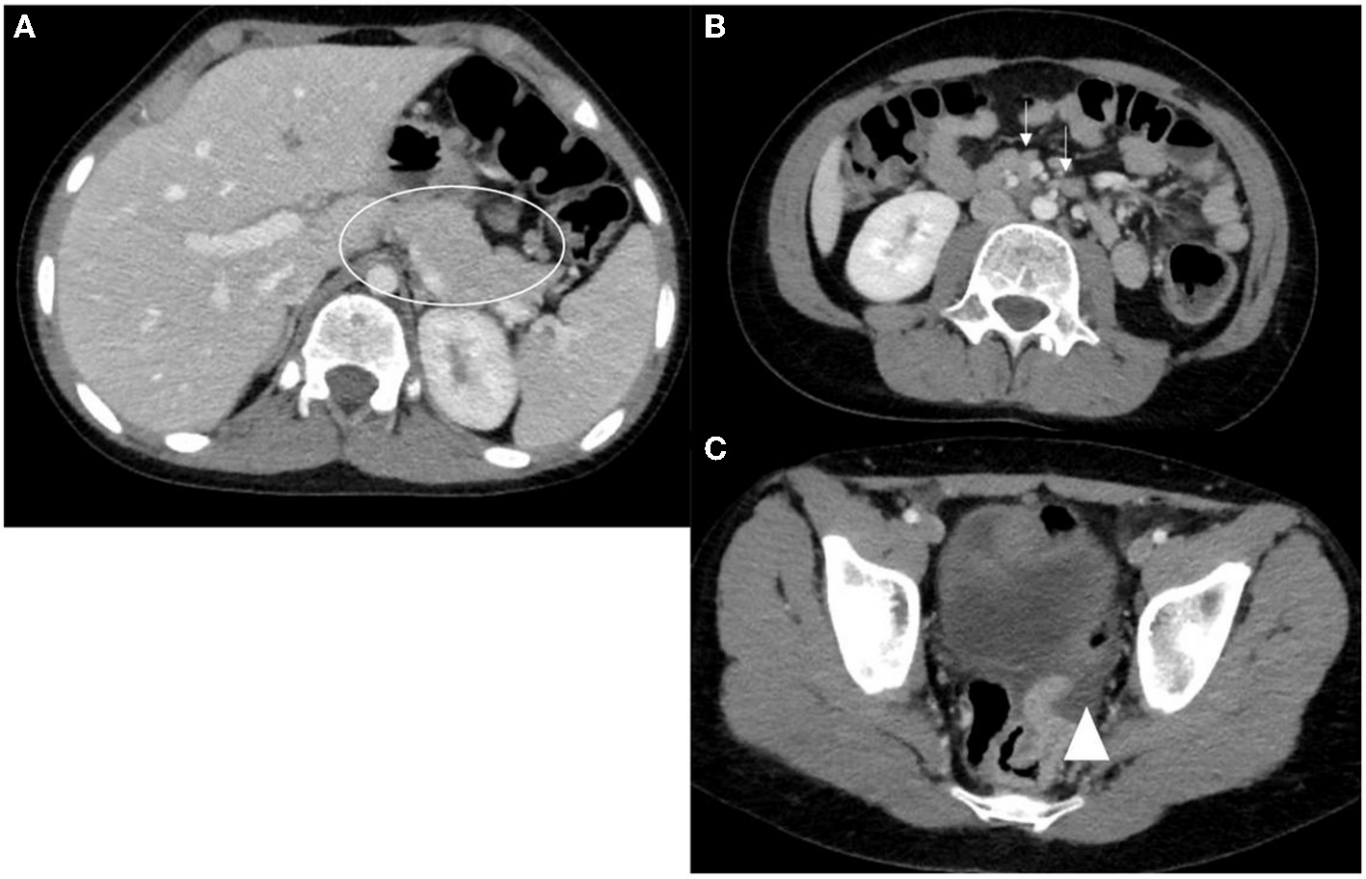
Axial CT scan images with oral and IV contrast. (A) Notice the bulky mildly heterogeneous pancreas (circle). (B) Multiple reactive peripancreatic lymph nodes (arrows). (C) Free fluid tracking down to the pelvis (arrow head).
